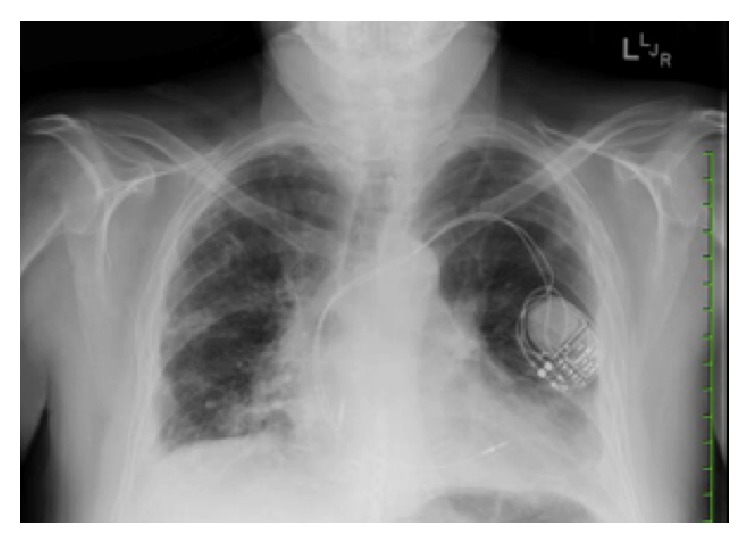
Chest radiography (CXR) showed diffuse patchy infiltrates and small bilateral pleural effusions.
